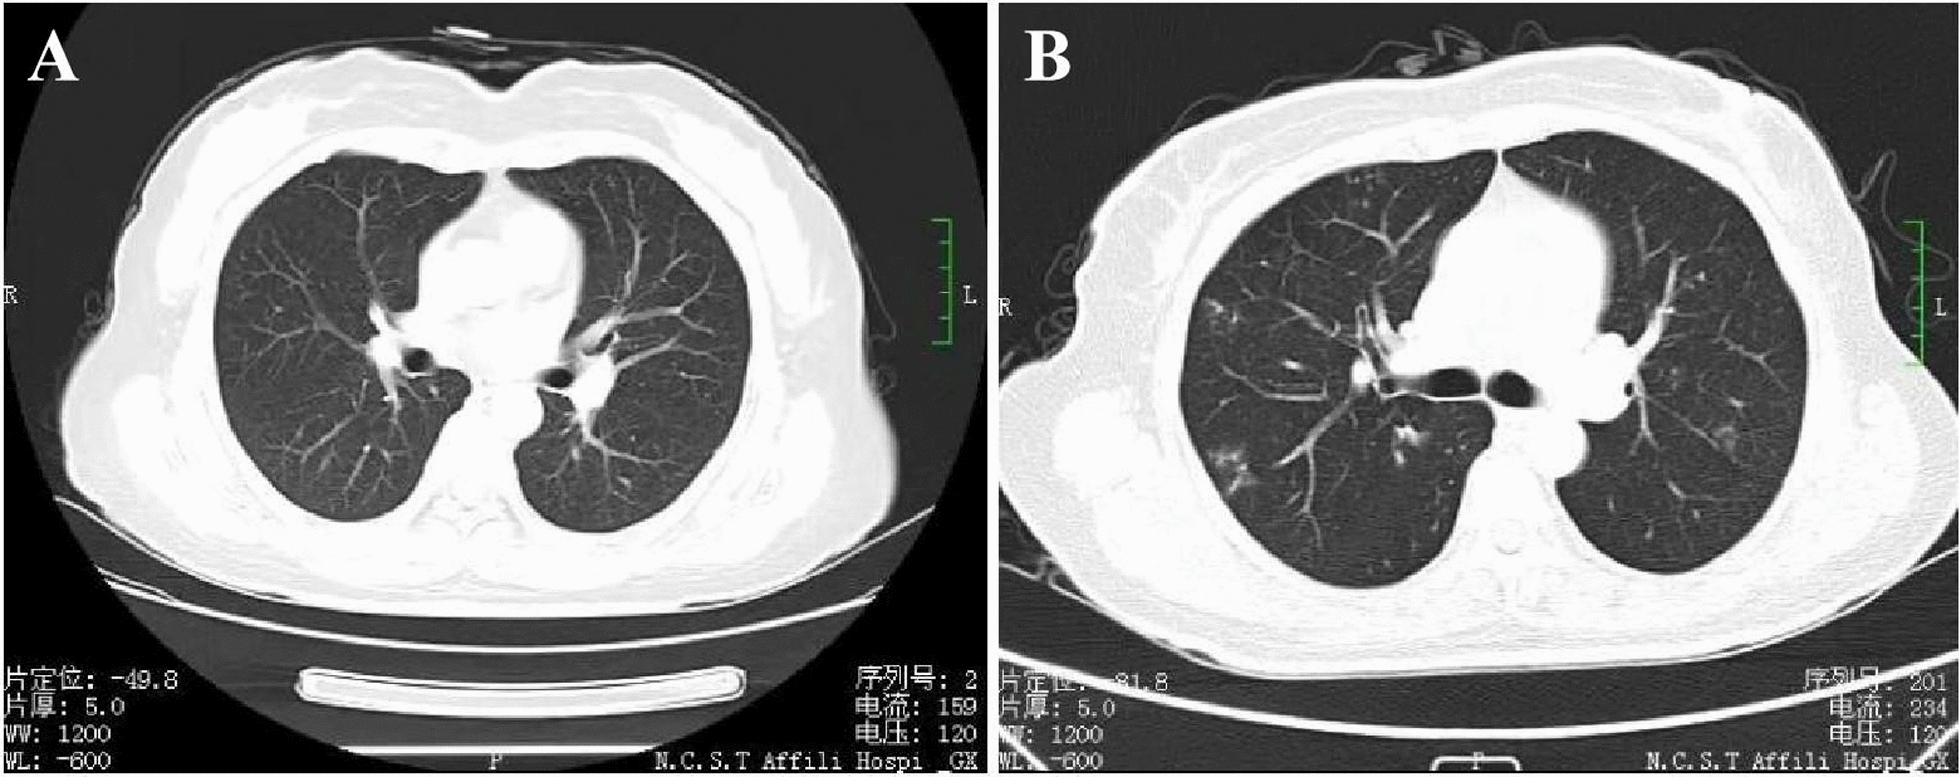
High-resolution computed tomography scans. A Example of no pulmonary interstitial changes in controls, show clear subpleural area and no reticular opacities. B Example of mild pneumonia in indium-exposed workers, show fine reticulonodular shadows. He had no history of cardiopulmonary disease and no abnormal findings onphysical examination. Results of health examinations were as follows: serum indium, 45.35 μg/L; KL-6, 6383 U/L; FVC, 84.4% predicted; FEV1/FVC, 115.19% predicted
